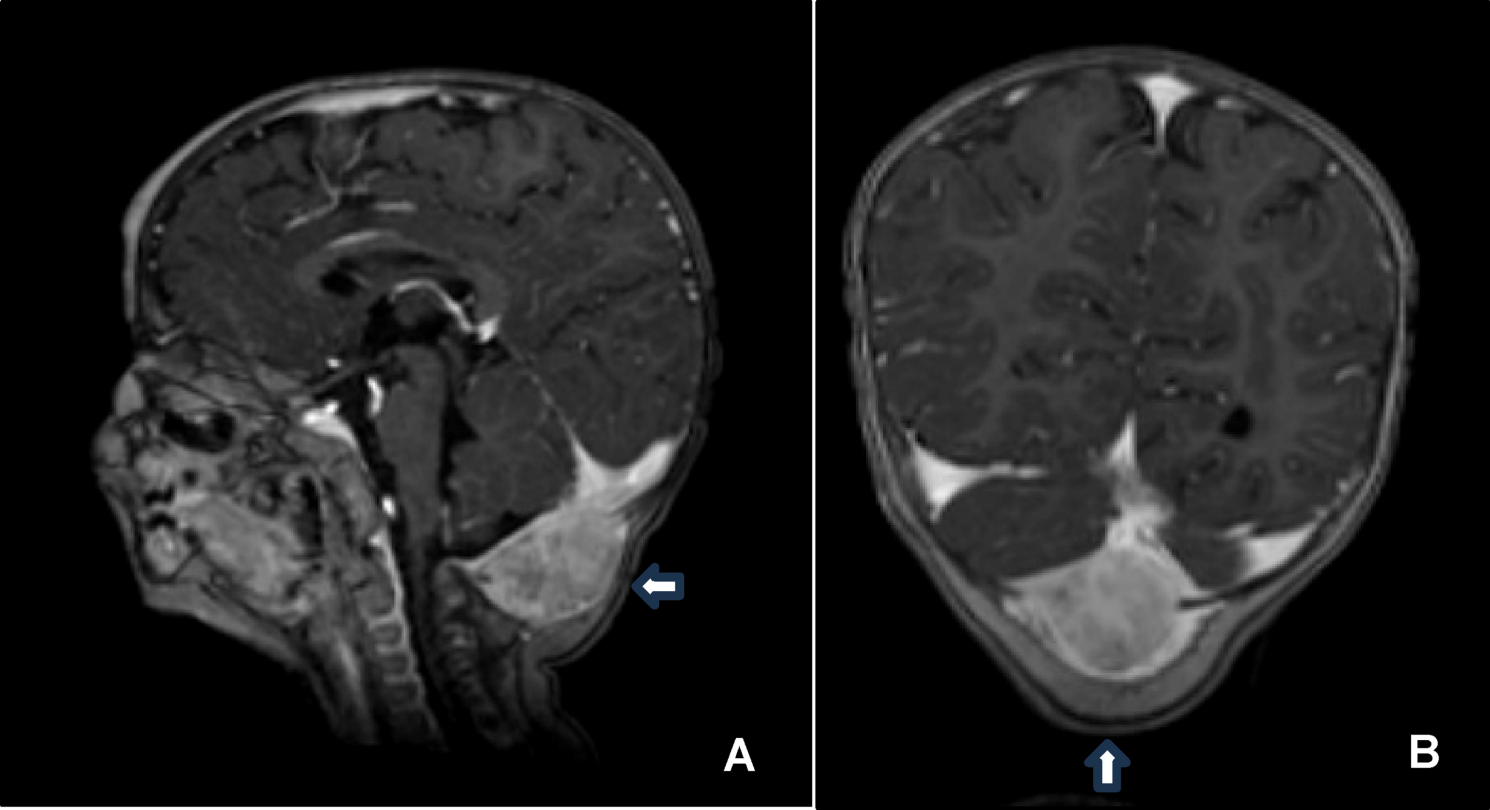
(A) and (B) Magnetic resonance imaging with postcontrast T1‐weighted sequence showing a locally aggressive expansive bone lesion in the occipital region, with evident enhancement and proximity to the torcula. There is no compressive effect on the structures of the posterior fossa.
